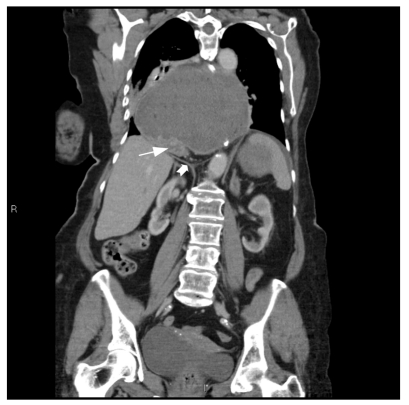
Coronal CT image demonstrates the duodenum compressed against the diaphragm. The distal body and antrum are distended with fluid and superior to the diaphragm. (White arrow: duodenum, Fat white arrow: diaphragm)
